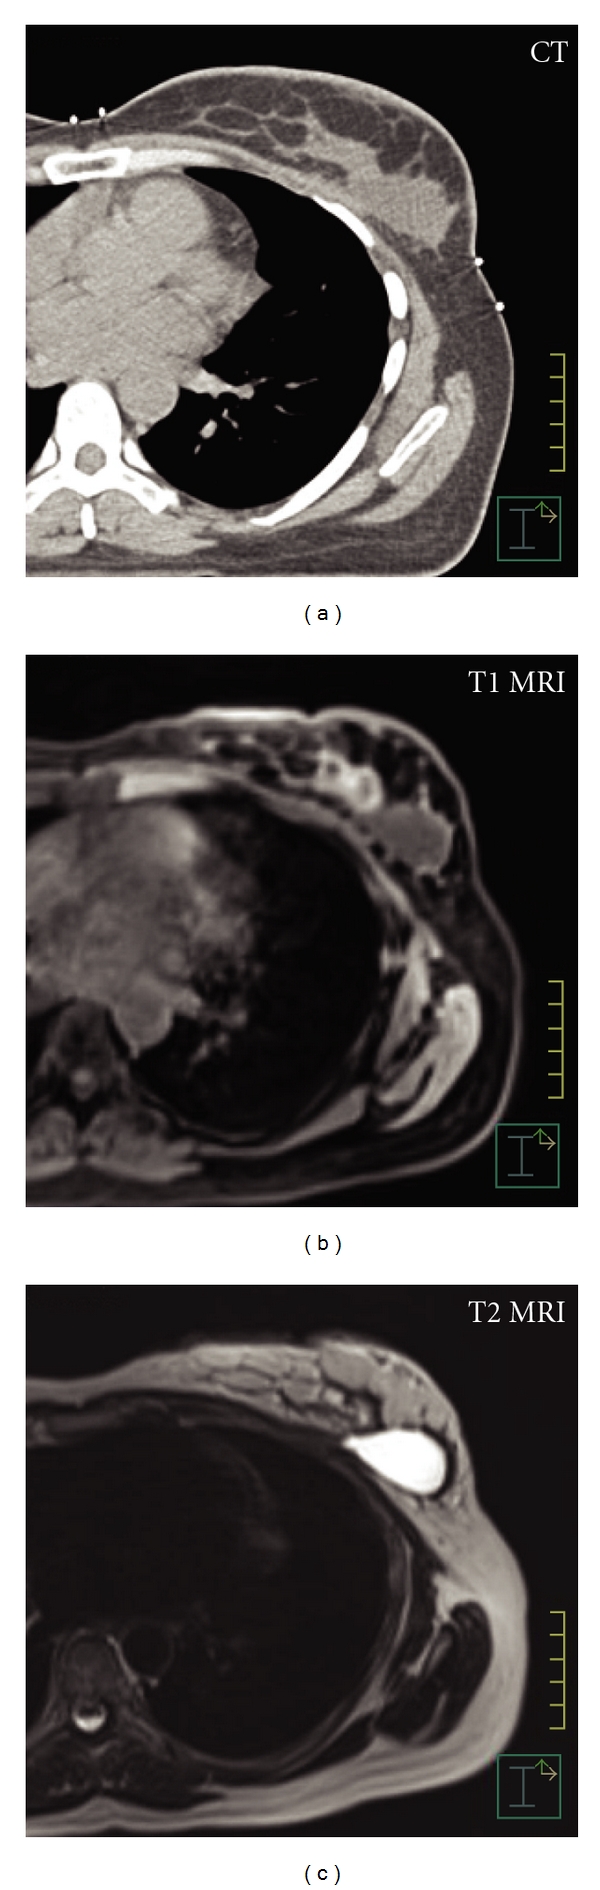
Comparison of imaging modalities. (a) CT shows homogeneous gray area. Borders are distinct laterally. Medially, the borders between lumpectomy cavity and breast parenchyma and chest wall are poorly defined. CVS 3. (b) T1 MRI, noncontrast, shows cavity with fairly well-defined borders, some rim enhancement. CVS 4. (c) T2 MRI noncontrast shows hyperintense signal consistent with seroma, distinct margins. CVS 5.
